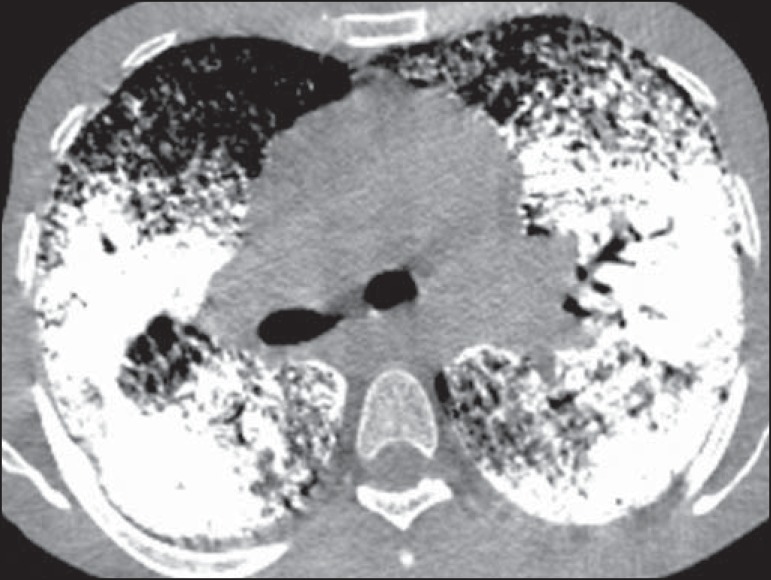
HRCT with mediastinal window demonstrating extensive consolidations with calcium
